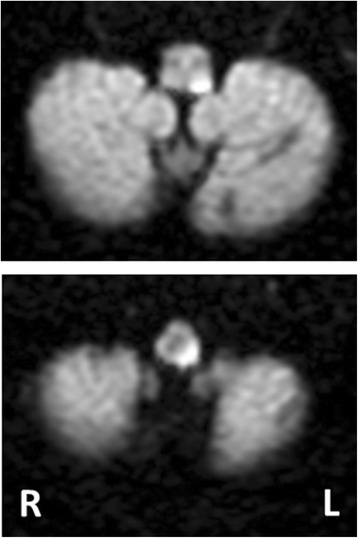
Brain magnetic resonance imaging of axial diffusion-weighted imaging demonstrating a high signal intensity lesion involving the lateral and dorsal medullae
